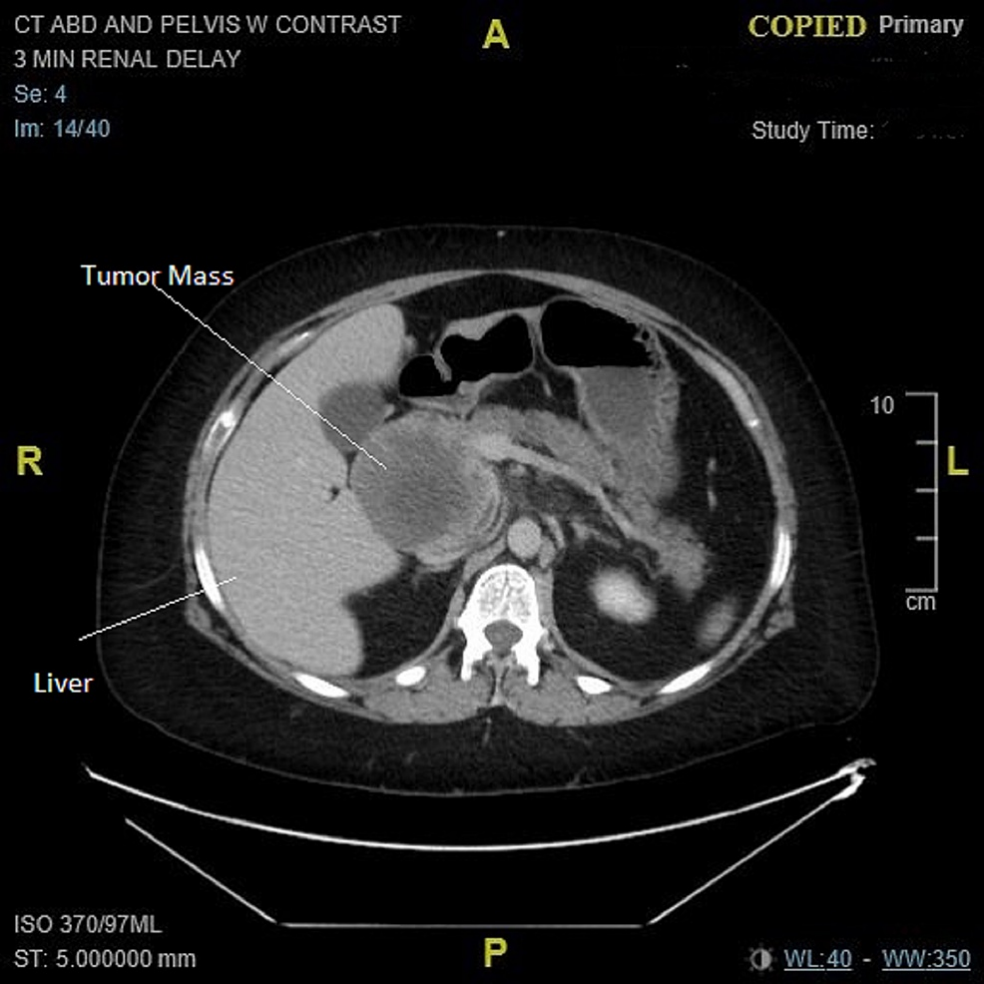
CT abdomen (axial plan): tumor mass in topography of head of the pancreas.
